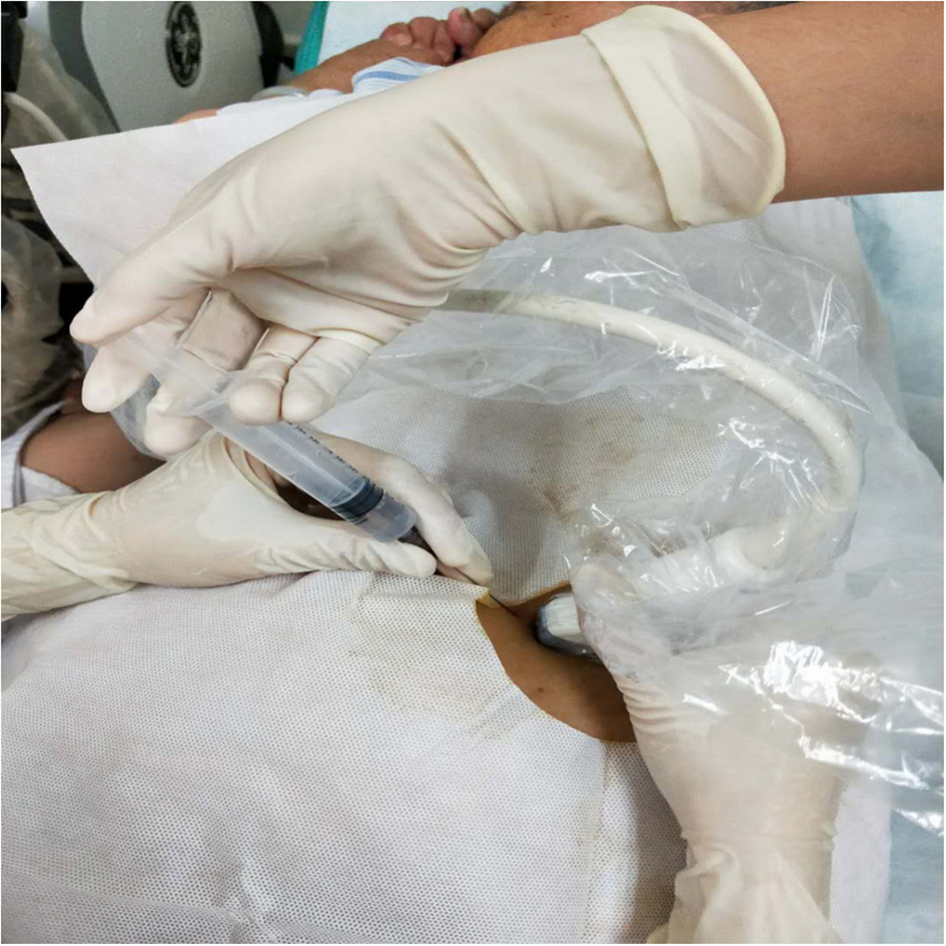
Ultrasound-guided injection of botulinum toxin A into the lateral abdominal muscles. EOM, external oblique muscle; IOM, internal oblique muscle; TM, transverse muscle.
